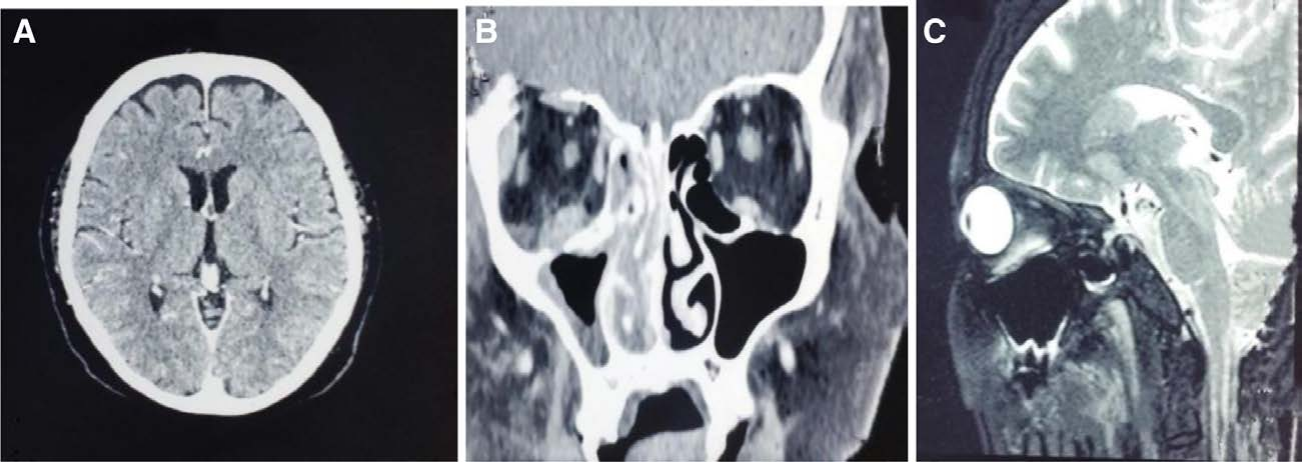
(A) Plain CT brain. (B and C) CT of PNS. CT = computed tomography, PNS = paranasal sinuses.
